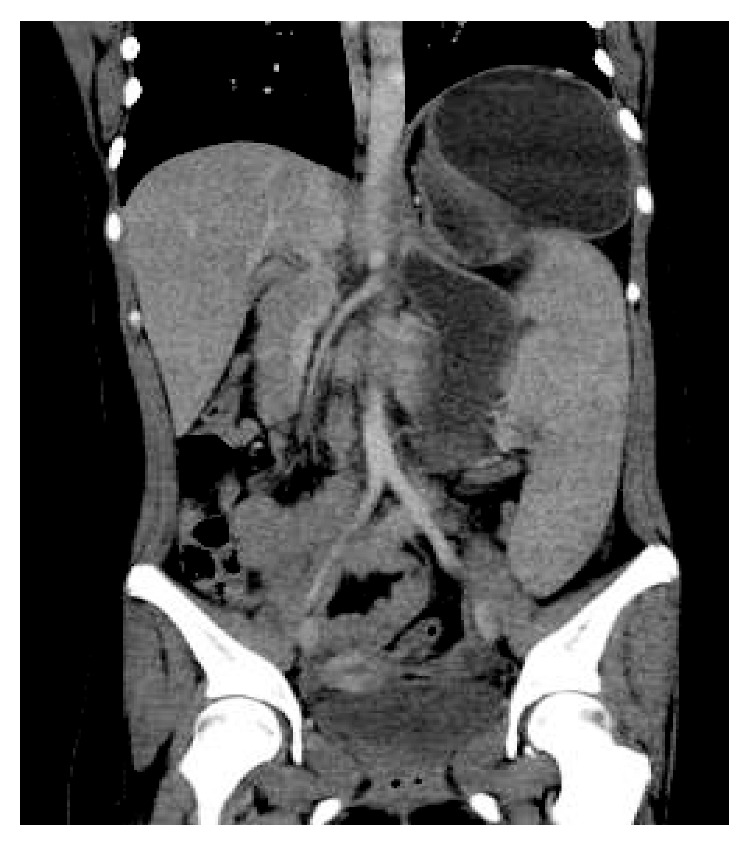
Coronal computed tomography scan of the abdomen showing marked gastric distension and splenomegaly.
